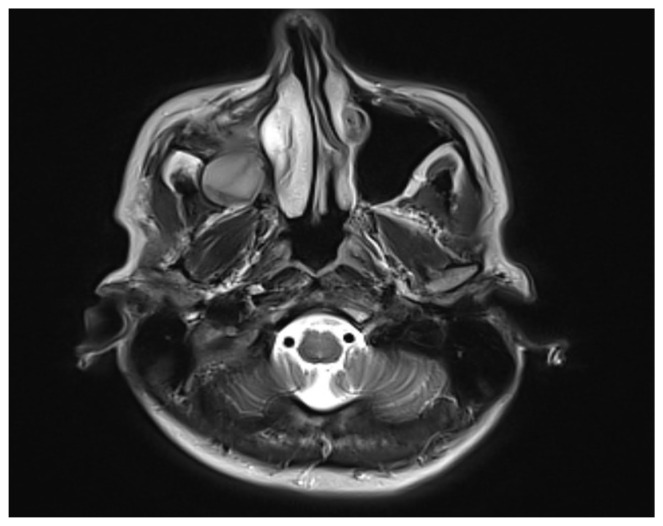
MRI scan of the head showing maxillary sinus cyst.
